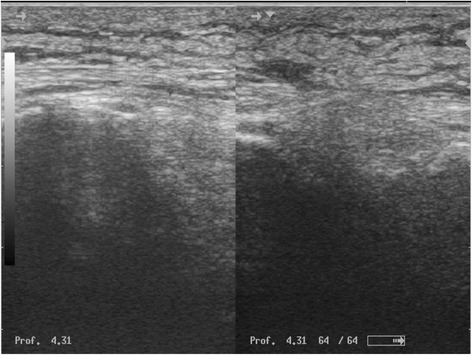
Ultrasound image of the soft parts of the leg. Thickening of the soft tissue with an echogenic laminated appearance
